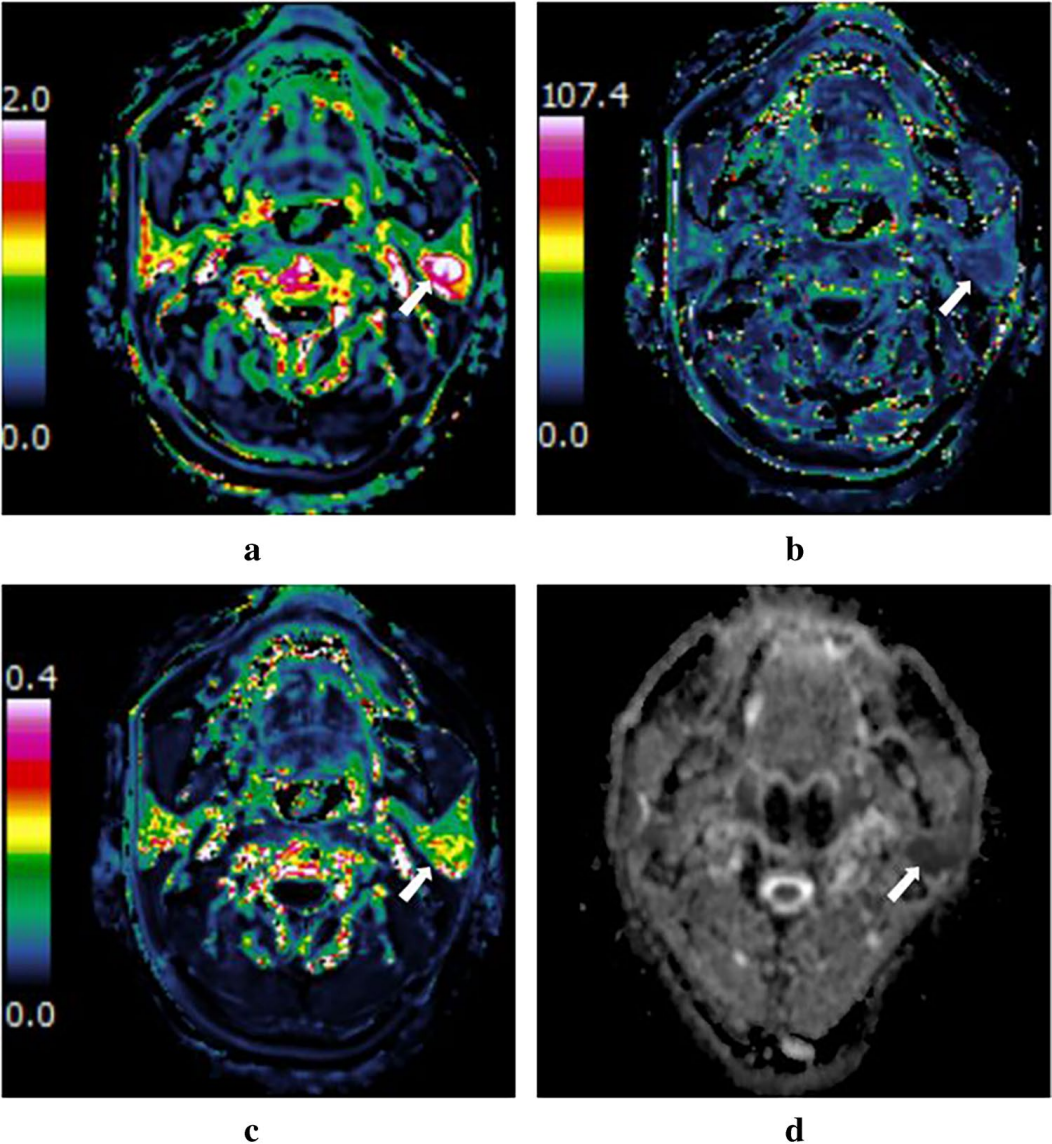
A 67-year-old man with Warthin tumor in the left parotid gland (white arrow). DCE-MRI parameter maps showed the *K*_ep_ (a), *V*_e_ (b), and *K*^trans^ (c) values of 2.02 min^−1^, 0.19, and 0.38 min^−1^, respectively. d
